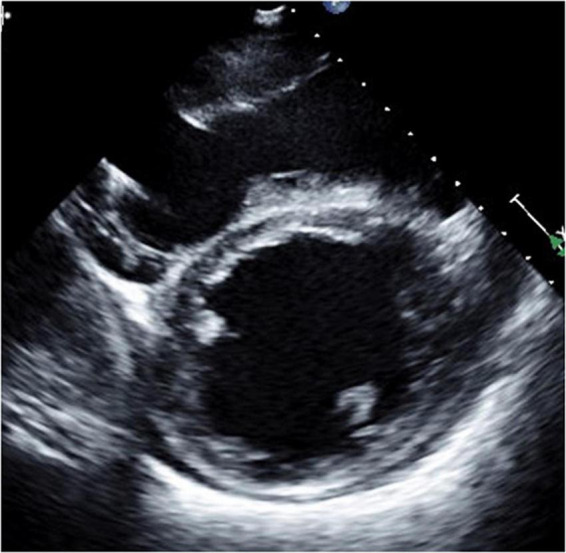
Short-axis view at end-diastolic phase of transthoracic echocardiography in Case 4.
